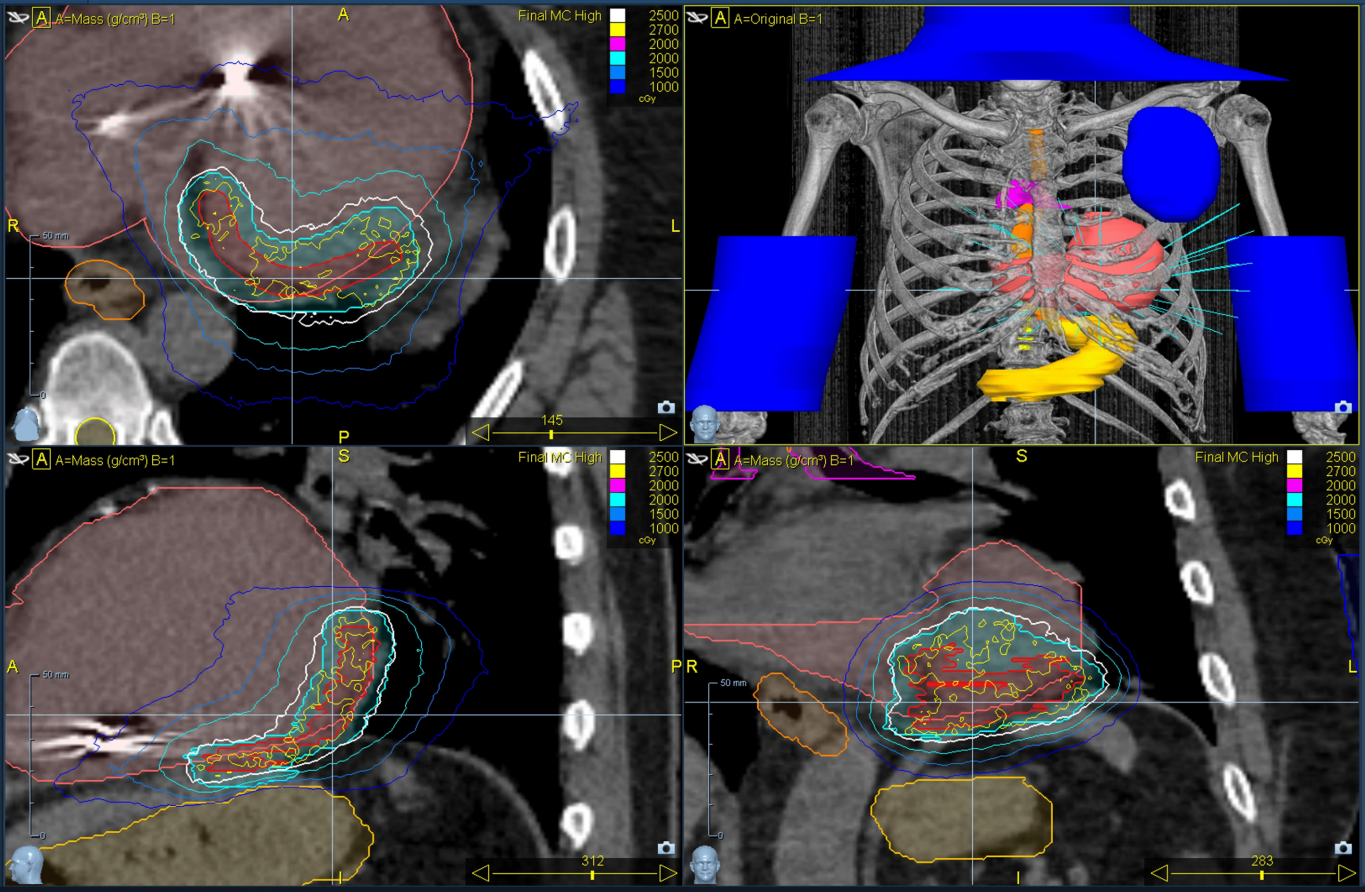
Cardiac SBRT target delineation and dose distribution The top left image shows an axial CT view of the heart, with the ICD located in the right ventricle and the arrhythmogenic area of the ventricle contoured, along with the dose distribution. The top right image is a 3D rendering that shows bony anatomy, including the organs at risk and the heart, contoured; the blue parts represent the contours used to block the entrance of the beam. Bottom left is a coronal view of the target contour, and bottom right is a sagittal view of the target contour, with dose distribution. SBRT: stereotactic body radiation therapy, CT: computed tomography, 3D: three dimensional
